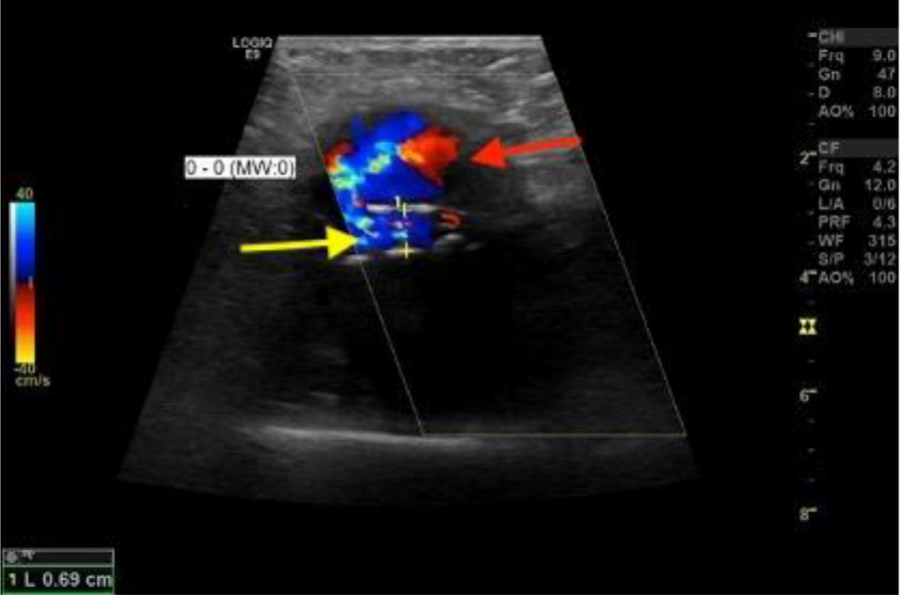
Duplex- Ultrasonography of the popliteal artery. Legend 4: Turbulent flow out of the popliteal artery, in the lower part (yellow arrow) of the popliteal artery (0.69 cm) and above it the extraluminal blood flow exiting through the injured vessel wall forming the pseudoaneurysm (marked with red arrow).
